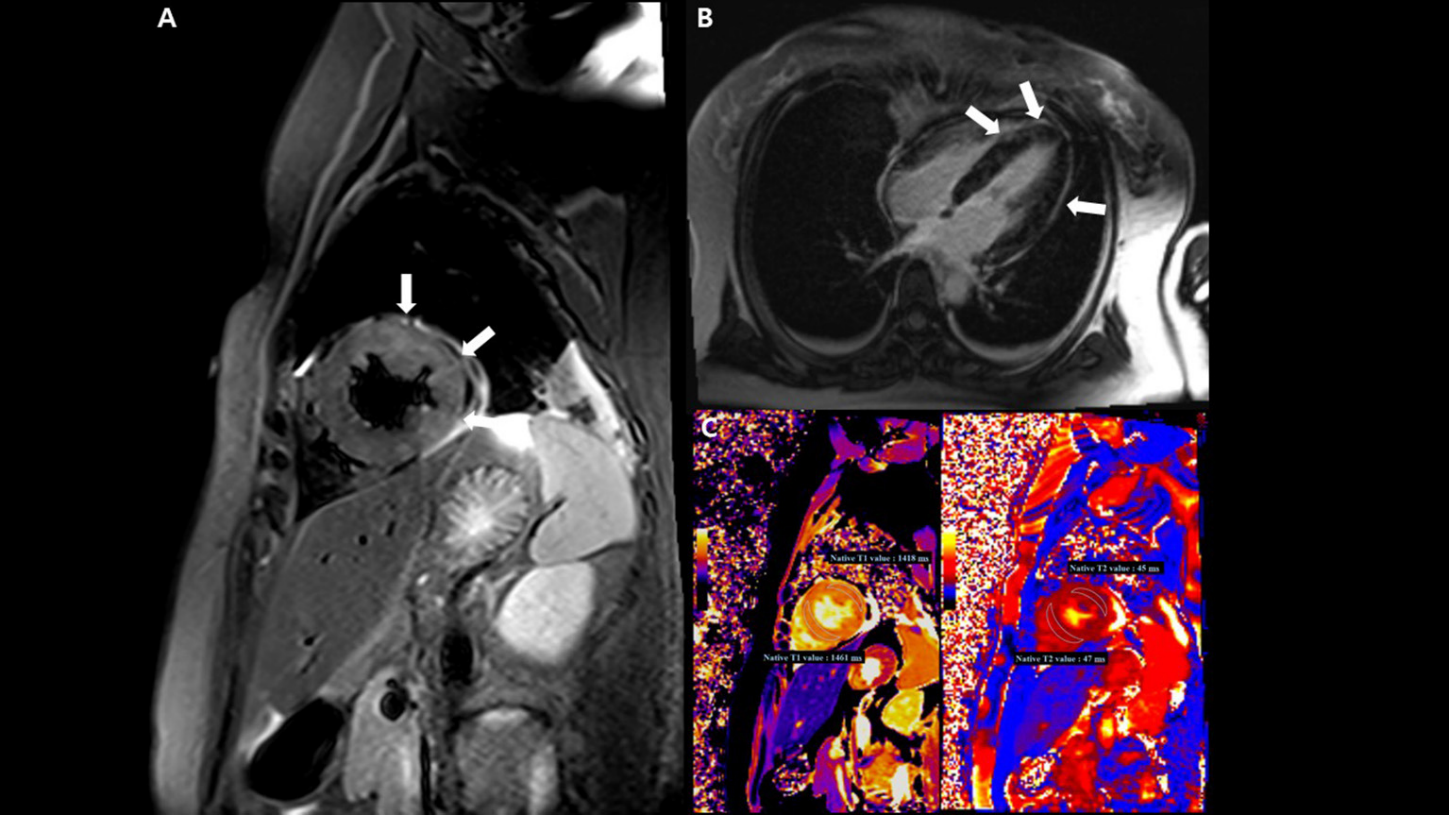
Cardiac magnetic resonance imaging (MRI) findings. (A) T2-weighted short TI inversion recovery MRI at 3T in a short-axis view shows multifocal high signal intensities at the mid anterior and lateral wall (arrow) indicating myocardial edema. (B) Late gadolinium enhancement imaging in a four-chamber view displays multifocal mid wall enhancement (arrow) indicating inflammatory myocardial necrosis. (C) T1 mapping and T2 mapping in a short-axis view show elevated T1 and T2 relaxation times at the mid ventricular level, indicating an acute myocardial injury (regional T1 relaxation time-1,418 ms at the anterolateral wall and 1,461 ms at the septal wall, regional T2 relaxation time-45 ms at the anterolateral wall and 47 ms at the septal wall; institution-specific cutoff values for acute myocarditis- T1 global: ≥1,230 ms and T2 global: ≥36 ms).
